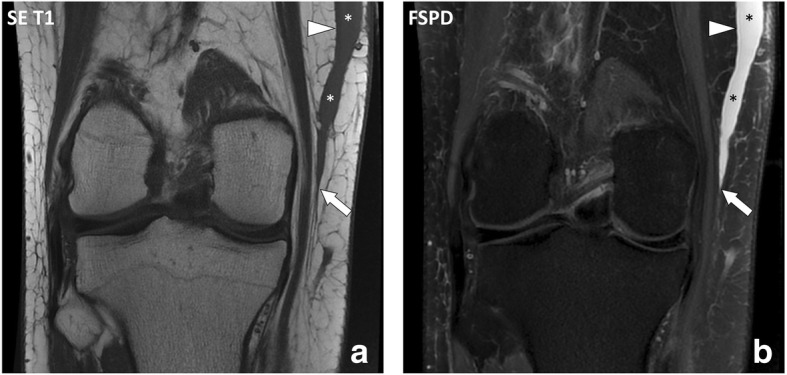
Coronal (a) SE T1w and (b) fat-suppressed proton density-weighted (FSPD) images of the right knee of a 23-year-old male with Morel-Lavallée lesion after a motorcycle accident. MRI demonstrates a fluid collection (asterisks) extending from the interface between the hypodermis and deep peripheral fascia (arrow) along the fascia superficialis (arrowhead)
